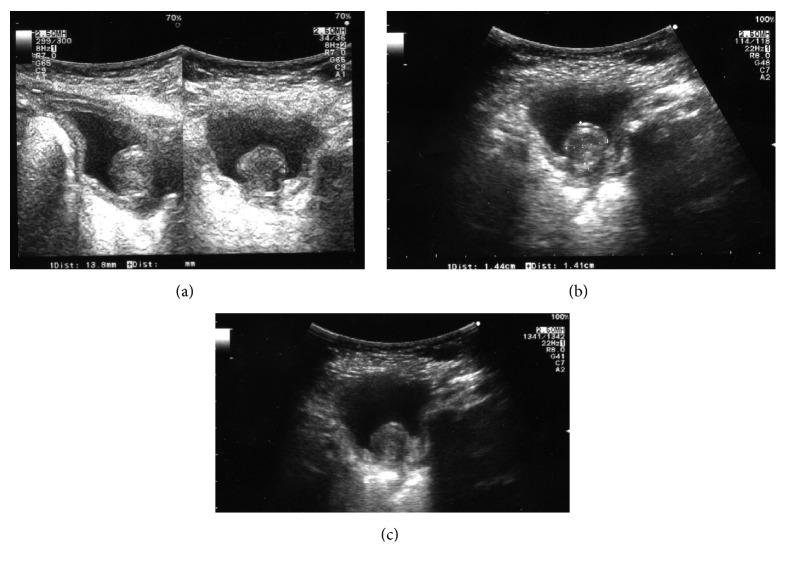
Ultrasound appearance of the mass: the solitary myofibroma arises from the bladder neck gaining the bladder lumen; (a–c): longitudinal and transverse US sections.
